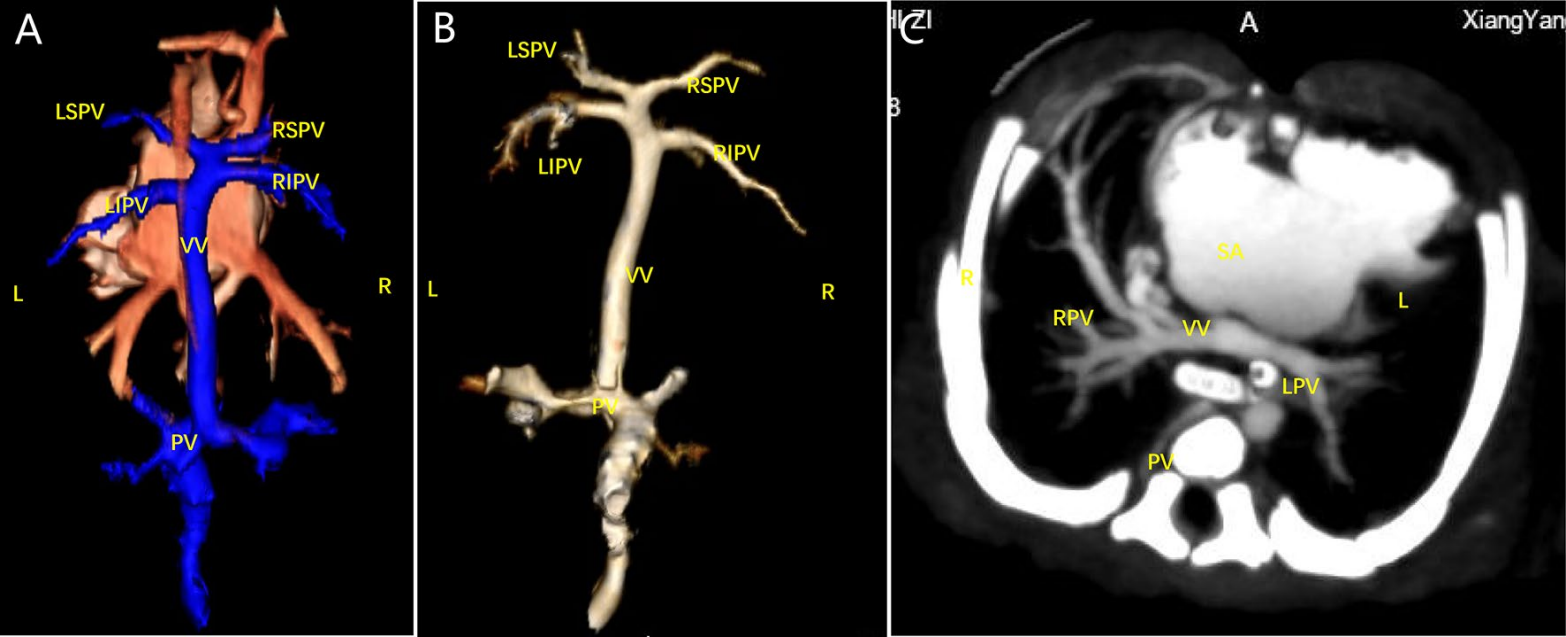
Postpartum CTA findings. (A–C) Left and right PVs converged to form a common PV trunk that flowed back to the portal vein via the vertical vein.
